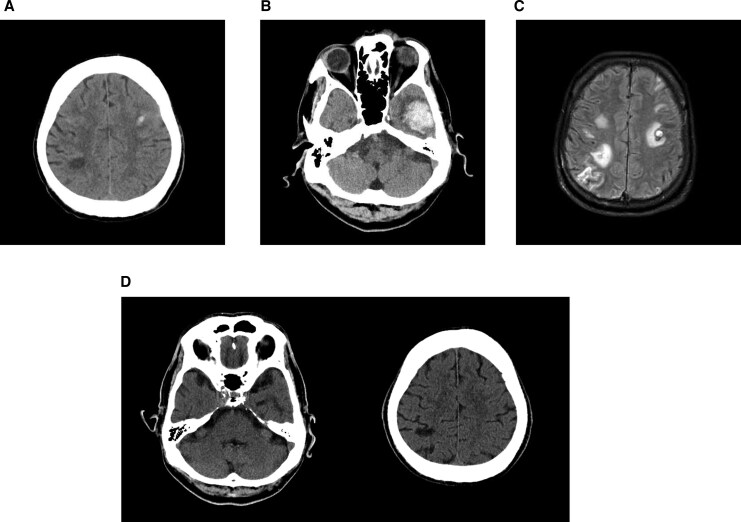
(*A*) Brain CT showed right parietal hypodensity and a small left frontoparietal intracerebral haemorrhage. (*B*) Repeat CT on follow-up revealed a new large left temporal lobe haemorrhage. (*C*) MRI (T2 fluid-attenuated inversion recovery (FLAIR)) demonstrated multiple rim-enhancing lesions. (*D*) Subsequent brain CT showed resolution of intracerebral haemorrhage.
